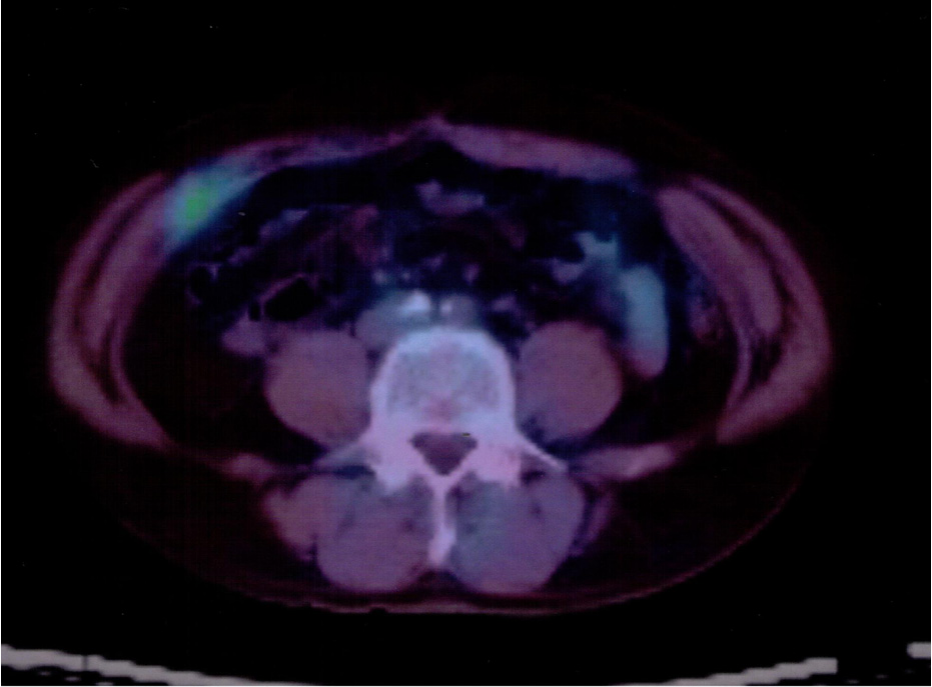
^18^F-fluorodeoxyglucose positron emission/computed tomography. ^18^F-fluorodeoxyglucose positron emission/computed tomography demonstrated increased ^18^F-fluorodeoxyglucose uptake (maximum standardized uptake value: 3.1) in the small nodule in the abdominal wall.
